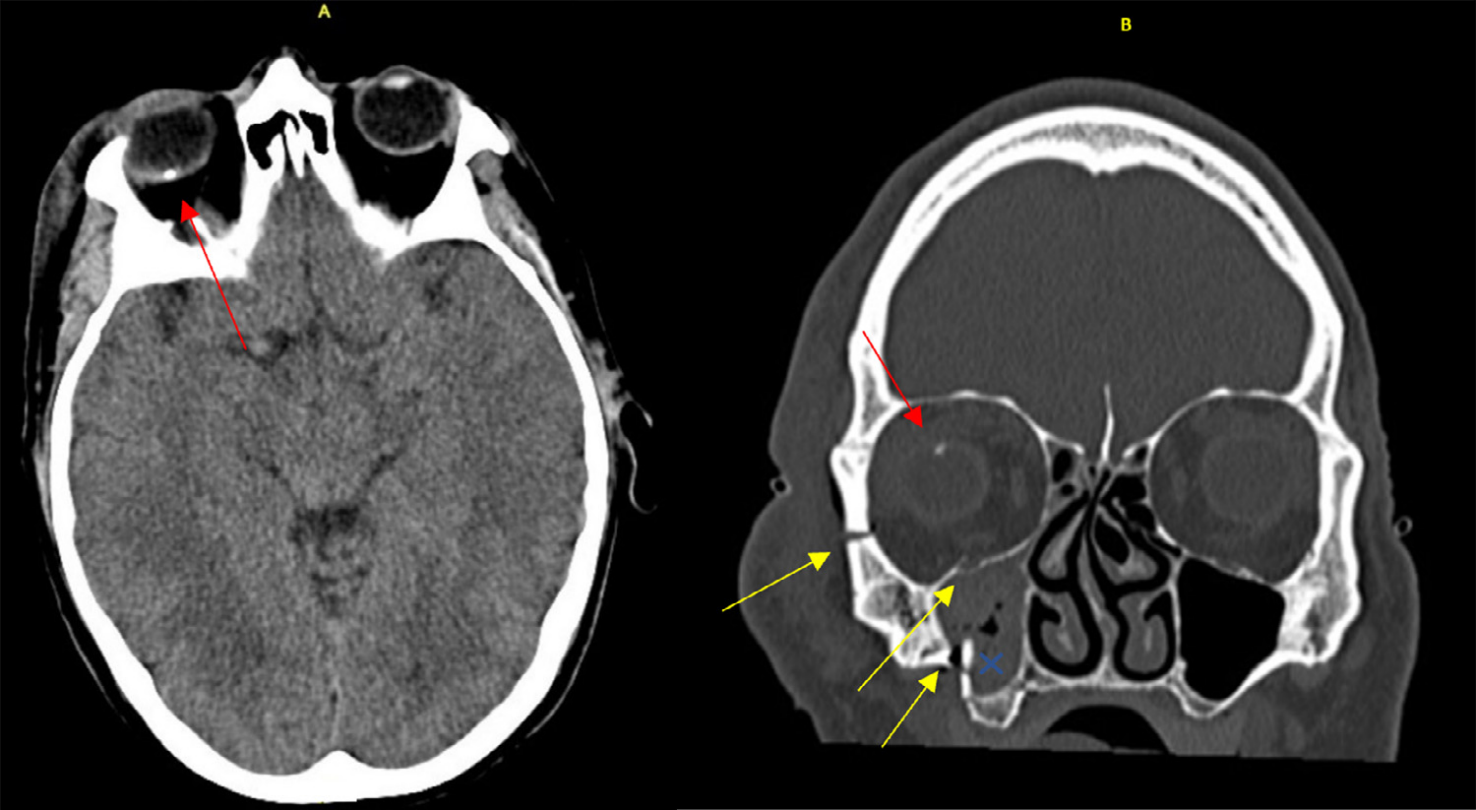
(A) Axial CT showing a hyperdense lesion in the right eye (red arrow). (B) CT scan of his face, which was done at the time of the accident, showed a right sided tripod fracture involving the right orbital floor, zygomatic arch and maxillary sinuses (yellow arrows). There is blood in the right maxillary sinus (x). A hyper-dense lesion is noted in the right eye on CT which raises the possibility of a retained IOFB (red arrow).
